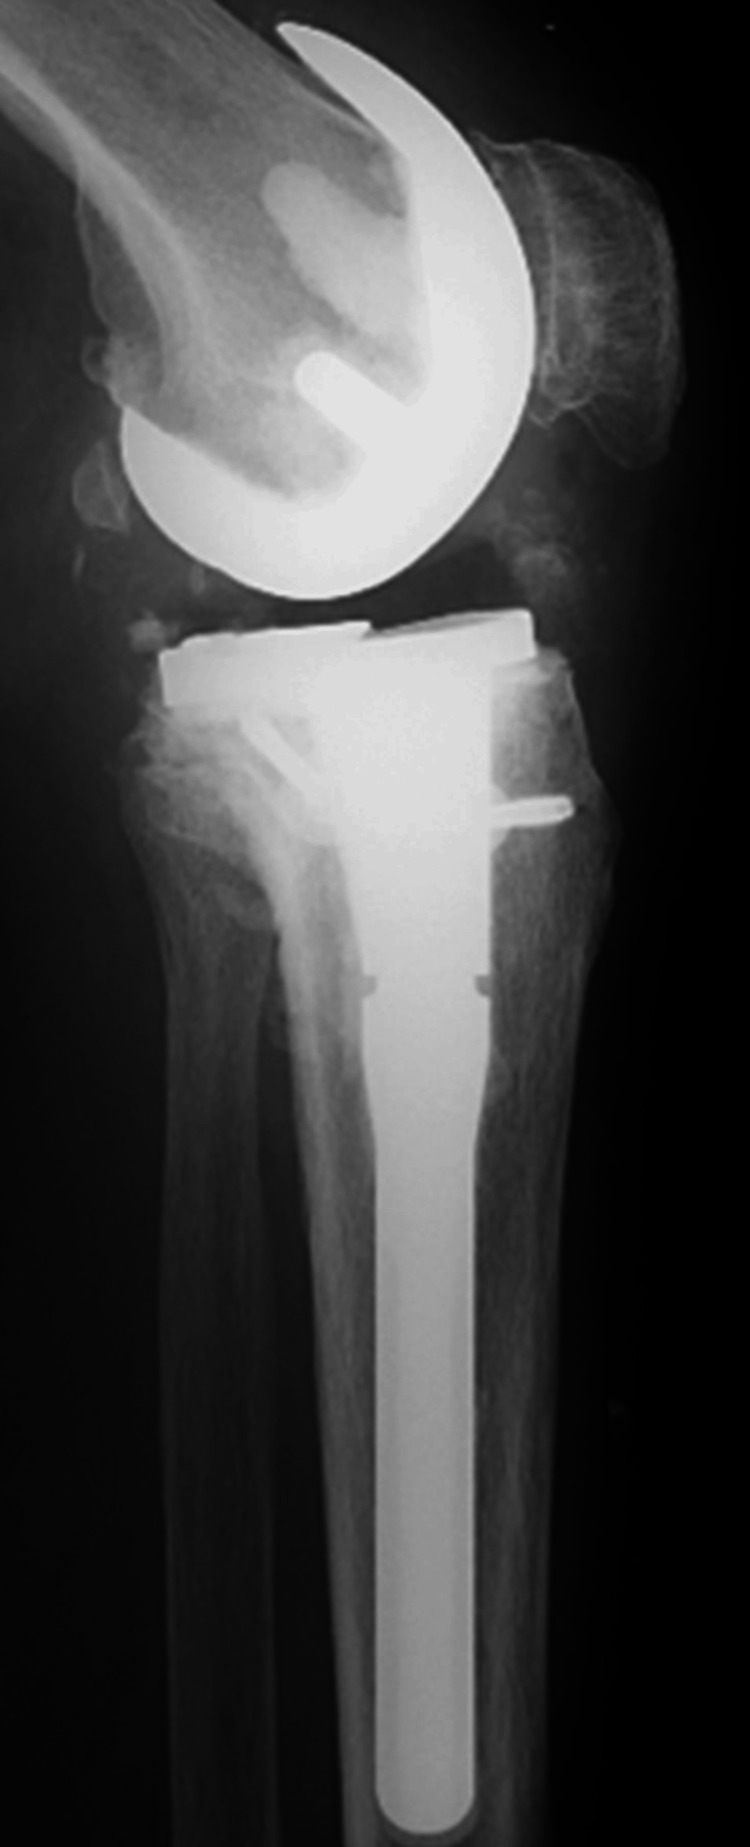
Immediate postoperative radiography of TKA (lateral view) TKA: total knee arthroplasty.
